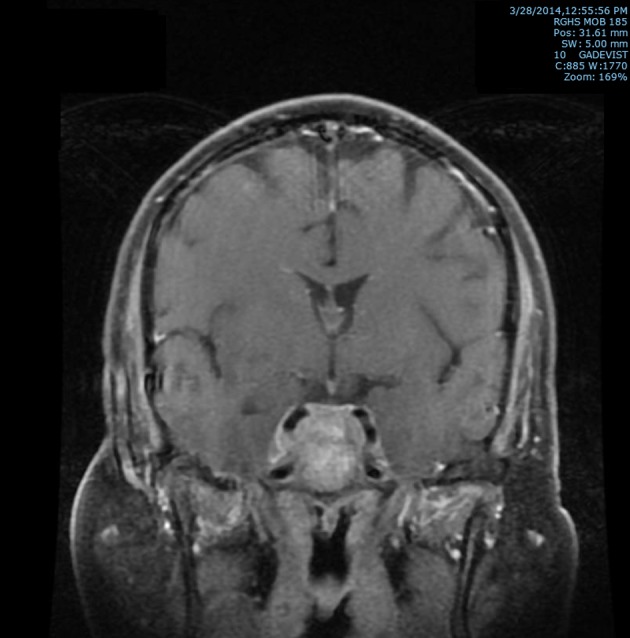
T2 post-contrast coronal MRI image of brain showing extension superiorly into the sella and posteriorly into the prepontine cistern with soft tissue prominence of right cavernous sinus with associated encasement of the right internal carotid artery.
